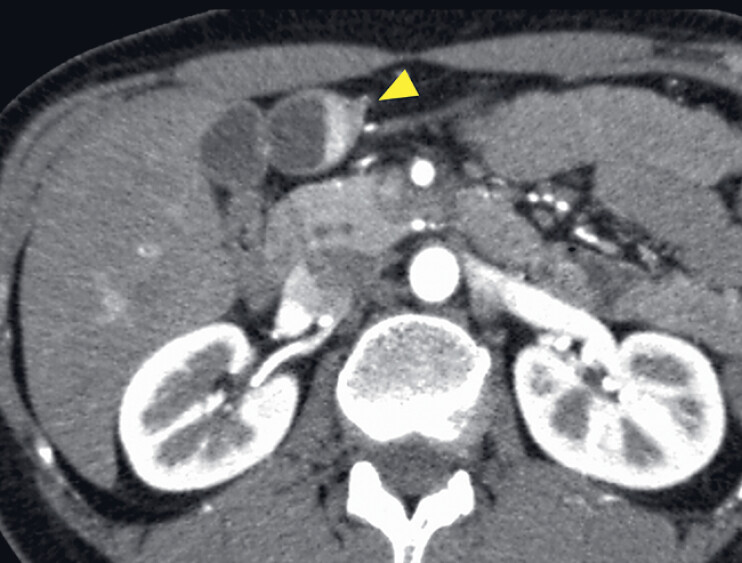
Contrast-enhanced computed tomography reveals a cystic lesion with an enhanced, partially thickened wall (yellow arrowhead) adjacent to the gallbladder.
